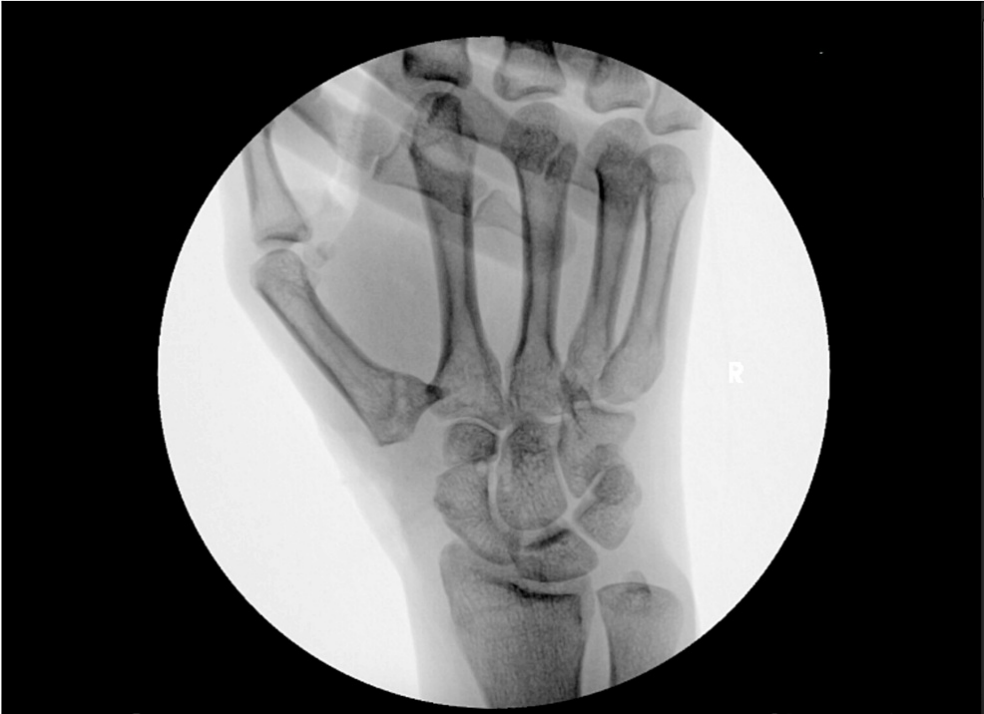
Intraoperative posteroanterior radiograph of the right hand demonstrating the absence of the trapezium after trapeziectomy with proper alignment of the first metacarpal.
